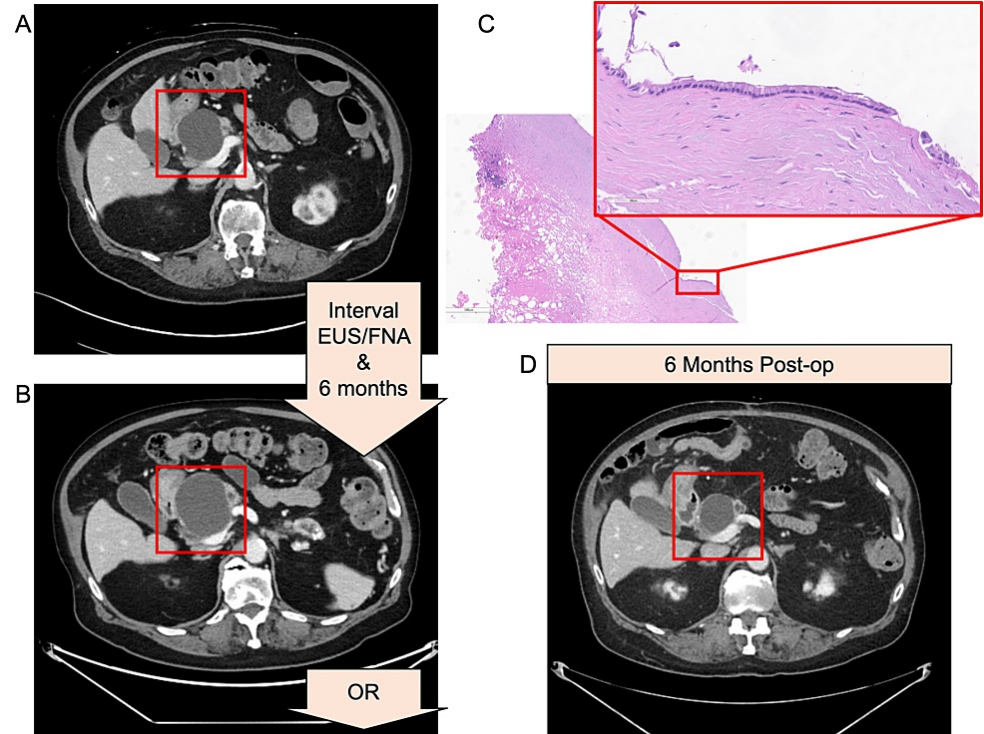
Radiographic and pathologic evidence. (A) The pancreas protocol CT that led to a referral to surgical oncology. (B) The six-month follow-up pancreas protocol CT demonstrated cyst growth. In these six months, the patient also had an EUS/FNA. (C) Operative cyst wall biopsy demonstrated a fibrotic cystic wall with focal residual epithelial lining. A representative high-power field shows mucinous epithelium with no high-grade dysplasia. (D) The six-month post-operative follow-up pancreas protocol CT demonstrated cyst shrinkage. EUS: endoscopic ultrasound, FNA: fine needle aspiration.
